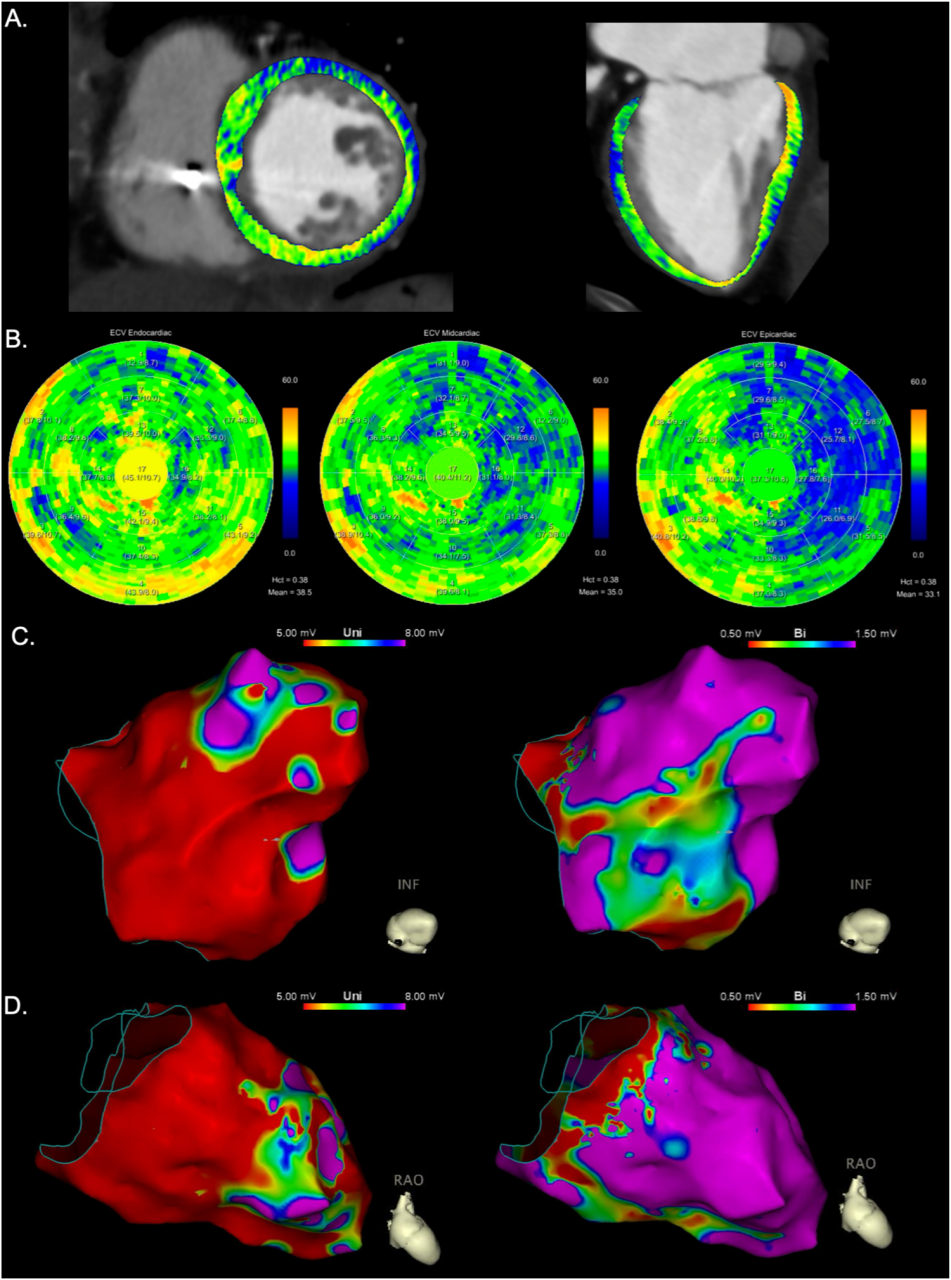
Representative images of the left ventricle of a 69-year-old female patient with dilatative cardiomyopathy. Coronary CT angiography with an overlay visualizing the extracellular volume (ECV) (A) and ECV polar maps (B) indicates diffuse fibrosis of the whole myocardium of the left ventricle. Polar maps suggest a fibrosis gradient from endocardial (inner 10% to 50% of the myocardium), to midcardiac (inner 25% to outer 25%), and epicardial (50% to outer 10%) layer. Endocardial electroanatomic mapping (EAM) (C, D) shows myocardial low-voltage areas of all left ventricular segments in unipolar (< 5.0 mV) EAM and low-voltage areas in the basal segments, as well as in the inferior segments in bipolar (< 0.5 mV) EAM
